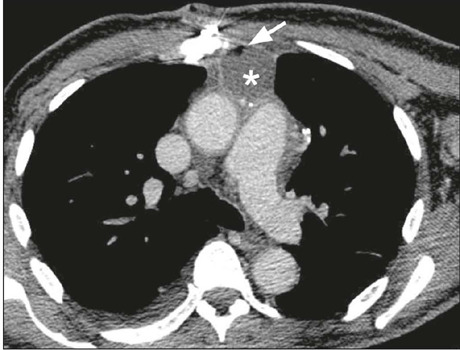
Retrosternal abscess (contrast-enhanced CT image; axial plane;
